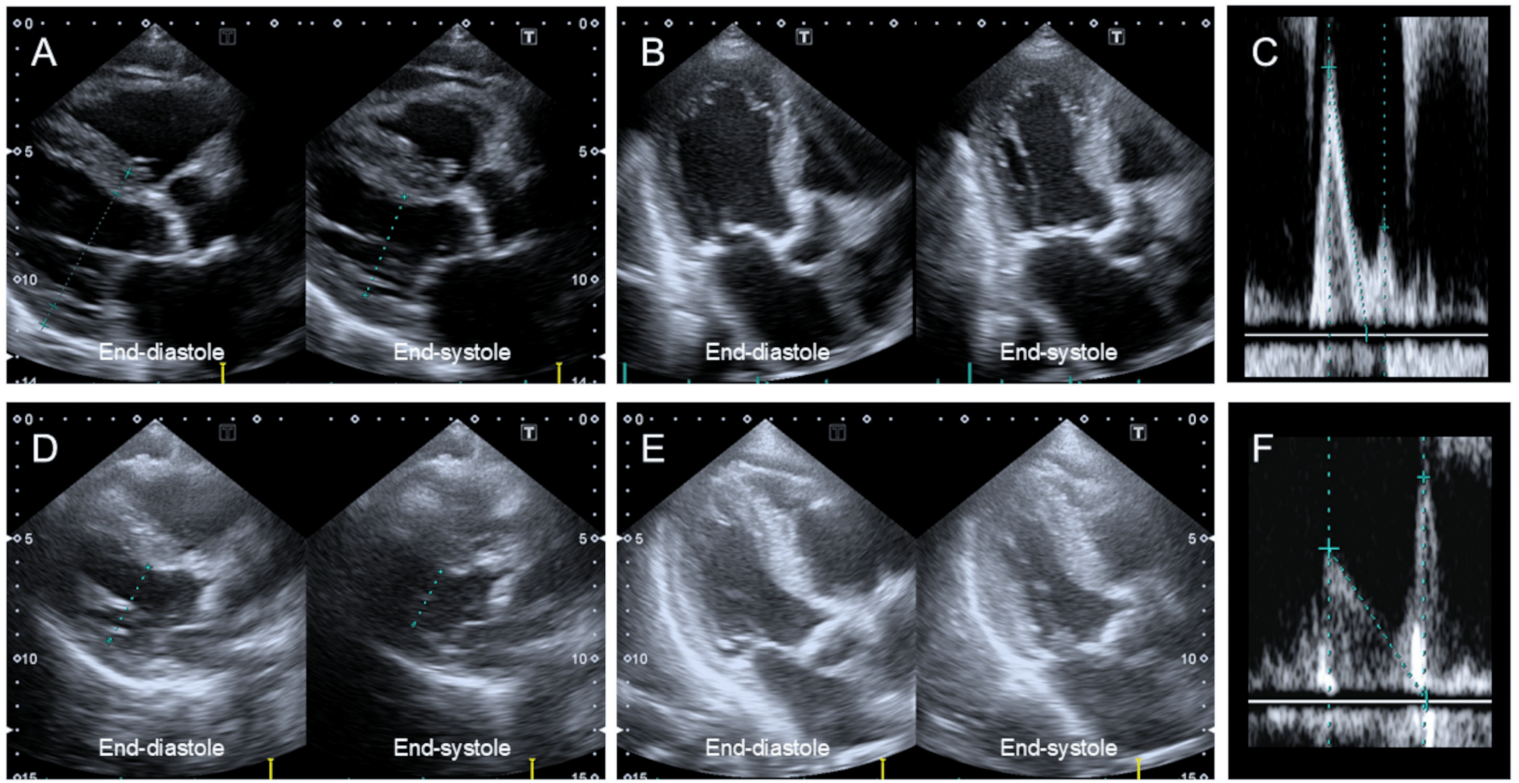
Transthoracic echocardiographic images A transthoracic echocardiography revealed a reduced EF of 35 % without LVH, with a LV end-diastolic diameter of 50 mm (A, B). Early to late diastolic mitral flow velocity ratio was 2.5, suggesting elevated left atrial pressure (C). After twelve weeks of conventional heart failure therapy, follow-up echocardiography revealed an improved EF of 55% (D, E), a decrease in LV end-diastolic diameter to 35 mm, and an early to late diastolic mitral flow velocity ratio of 0.7 (F), confirming the diagnosis of HFimpEF and indicating LV reverse remodeling and optimized volume status.
